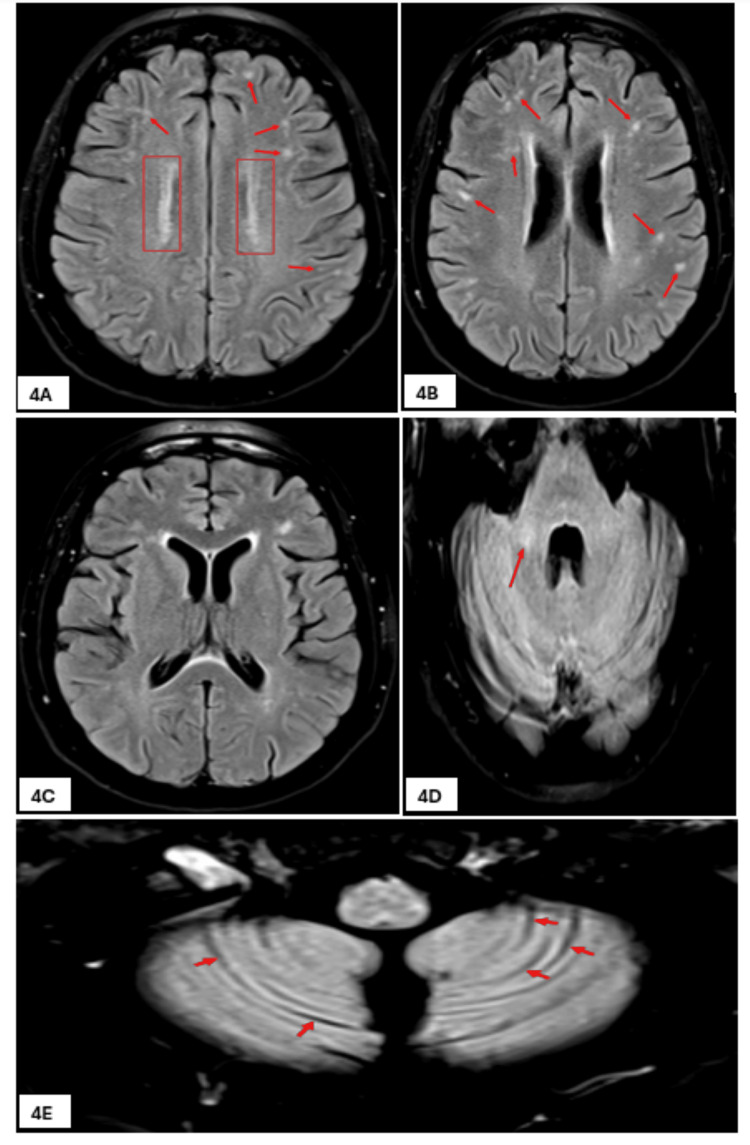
Third MRI scan of the brain. Two-dimensional axial fluid-attenuated inversion recovery (FLAIR) images showing progressive development of bilateral subcortical foci (arrows in 4A and 4B) and deep periventricular white matter sheets of FLAIR signal elevation (rectangle in 4A), with the latter showing predilection for a perimedullary venous location (4A and 4B compared with 1A, 2A, and 3A), with resolution of the basal ganglia foci (4C compared with 3B) as well as tegmental pontine signal alteration (4D dcompared with 3C), and interval development of a right middle cerebellar peduncle ill-defined focus of FLAIR signal alteration (arrow in 4D compared with 3C), and development of early cerebellar atrophic changes (arrows in 4E compared with 1D).
